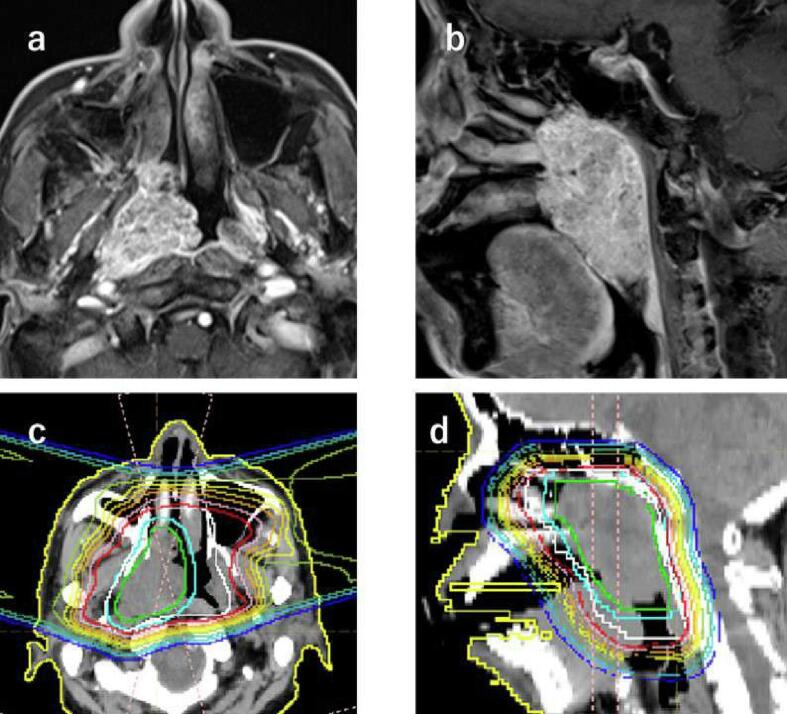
Contrast-enhanced T1-weighted MRI of a nasopharyngeal tumor and treatment planning for PBT. (a) Axial section of contrast-enhanced T1-weighted MRI. (b) Sagittal section of contrast-enhanced T1-weighted MRI. (c) Axial section for treatment planning. (d) Sagittal section for treatment planning.
